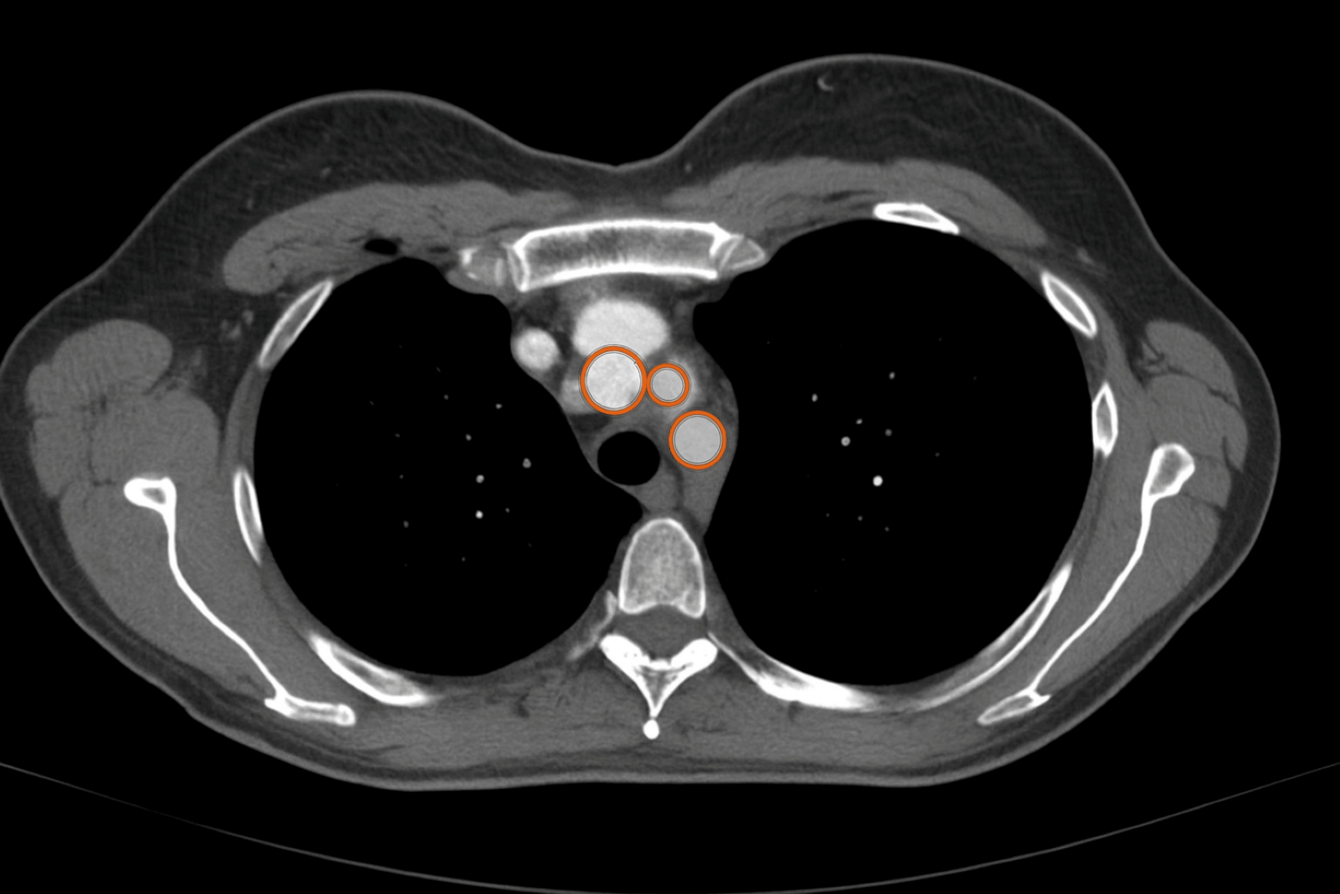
Axial contrast-enhanced CT chest demonstrating diffuse concentric mural thickening of the thoracic aorta (circled in red), most prominent in the ascending and descending segments. These findings are highly suggestive of inflammatory aortitis, consistent with large vessel vasculitis (LVV). This imaging played a pivotal role in prompting further vascular evaluation.
